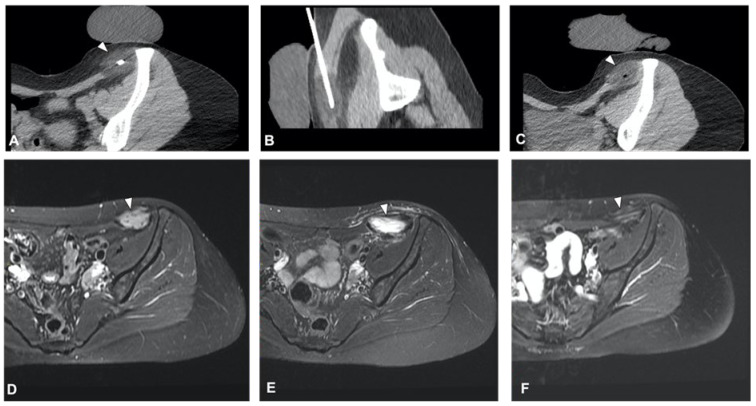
A 46-year-old patient with desmoid tumor of the abdominal wall showed in axial T2 fat sat MR image ((D)—arrowhead). Axial (A) and sagittal oblique (B) CT images showing the cryoprobe inside the lesion and the post-procedure ice ball containing the whole lesion (C). Axial T2 fat sat MR image 3 months after treatment shows an increase in T2 signal inside the lesion, suggesting necrosis (E), and the control with 6 months shows no residual tumor (F).
